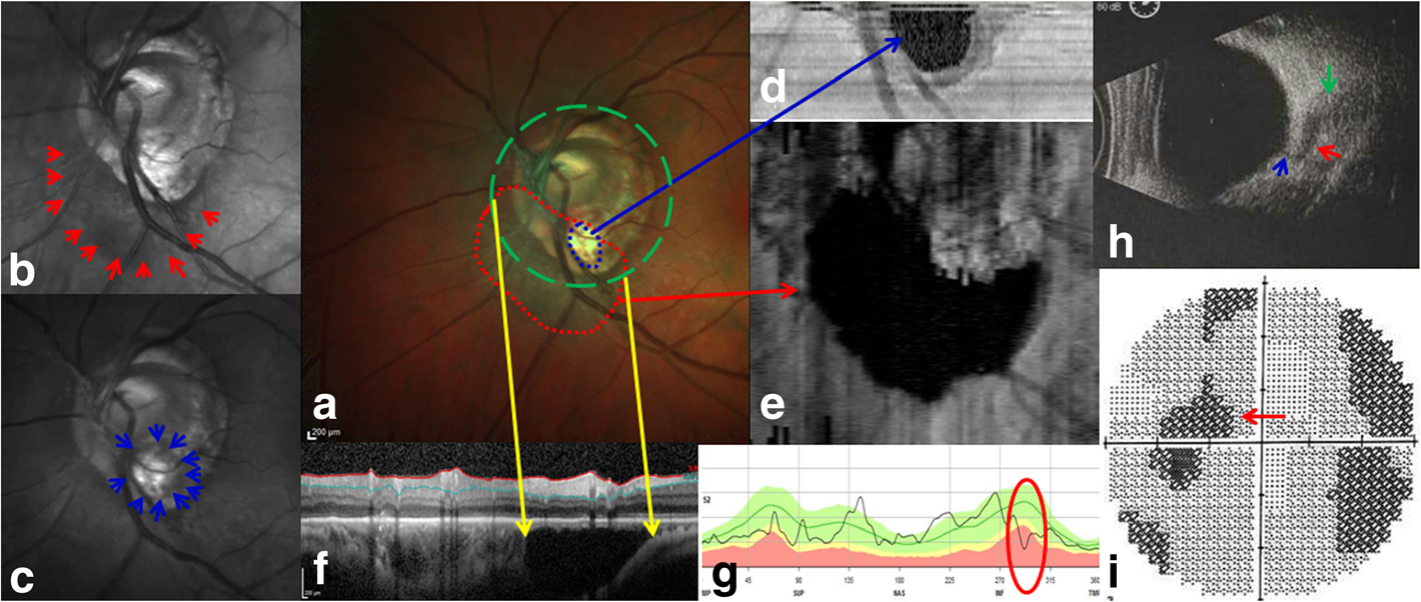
Identification of peripapillary intrachoroidal cavitation and sinkhole on multi-modality images. a Multi-color images of peripapillary intrachoroidal cavitation and tilted ONH show a well-circumscribed, caesious, peripapillary lesion coalesced with the ONH (red dots) and its inferotemporal sinkhole (blue dots) in the myopic conus. The inferotemporal retinal vein covers the sinkhole of the peripapillary intrachoroidal cavitation in the myopic conus. b Infrared reflectance demonstrating a peripapillary dark reflective region (red arrows), corresponding to the red dots in (a, c). A clear border of the sinkhole (blue arrows) could be observed in green reflectance, corresponding to the blue dots in (a, d). The sinkhole (size: 0.34 mm^2^) on an *en face* image (blue arrow). e The intrachoroidal cavity (size: 7.85 mm^2^) on an *en face* image (red arrow). f EDI-OCT (green dashed circle in a) showing the entire profile of the peripapillary intrachoroidal cavitation (yellow arrows). g A thinner retinal nerve fiber was detected above the peripapillary intrachoroidal cavitation region (red circle), in accordance with the EDI-OCT image in (f, h). The coalescence of peripapillary intrachoroidal cavitation (red arrow) and ONH (green arrow) as evident on an ocular B scan ultrasound image, as well as the sinkhole (blue arrow), consistent with (a). i Visual field testing shows that the dark defects (red arrow) connected with the blind spot, consistent with the peripapillary intrachoroidal cavitation location in (a) and (h). (ONH, optic nerve head; EDI-OCT, enhanced depth imaging optical coherence tomography)
